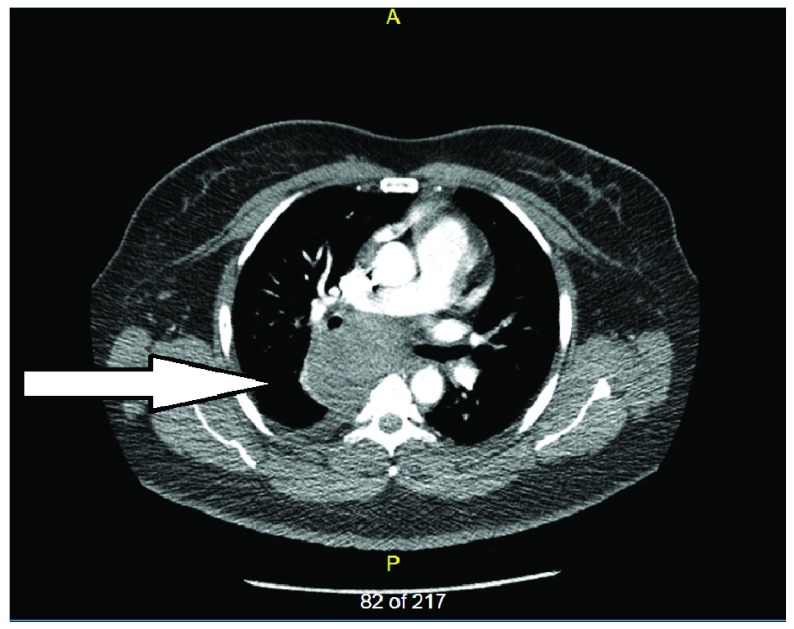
CT Chest with contrast showing right posterior mediastinal cyst measuring 9.64 X 7.7 cm (April 2017).
